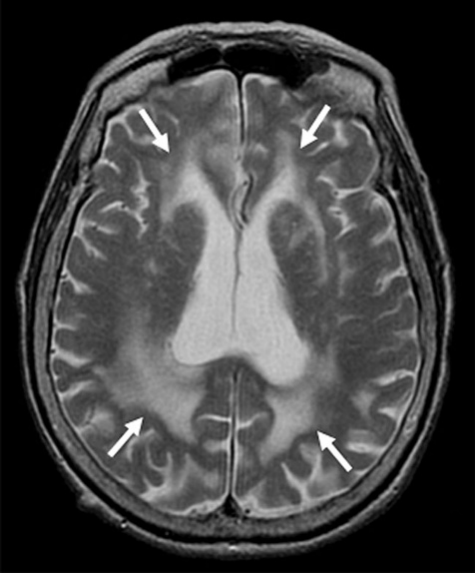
Leukoaraiosis in an 87-year-old woman with pathologically proved CAA and AD. Axial T2-weighted images showed bilateral hyperintensities (*arrows*), which involved the posterior dominant periventricular and deep white matters
